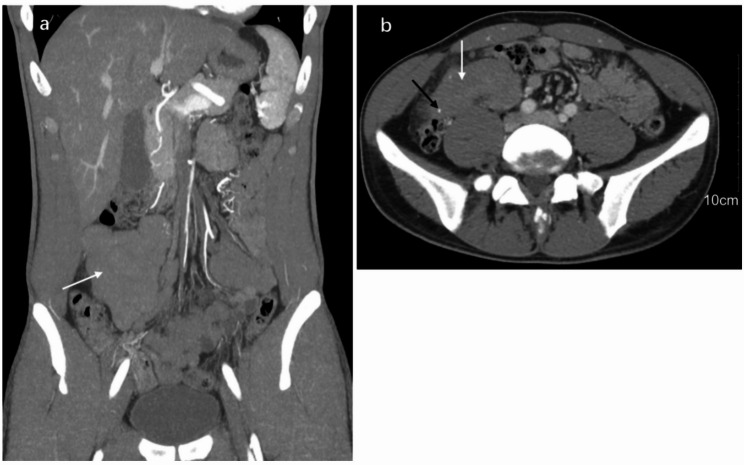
CT image of RNFP. The irregular masses were partially fused (white arrow), with calcifications(black arrow), and exhibited progressively mild enhancement, with minimal enhancement in the arterial phase and gradually increased enhancement in the portal venous and delayed phases (a, b)
